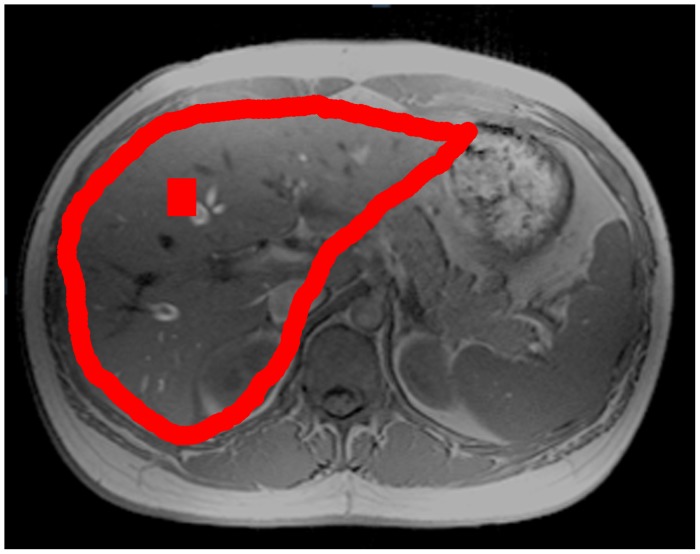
T1-weighted MR image indicates the spatial localization of EPSI plane. ROI used for the calculation of HFC over total cross section of liver was shown on the image.
